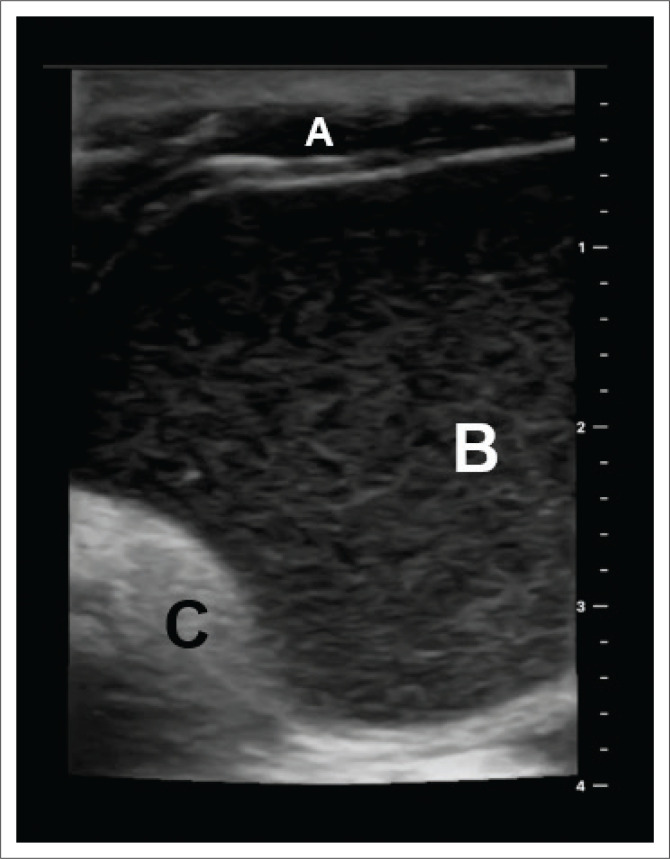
Pre-operative ultrasound imaging. The top of the image is superficial, whereas the bottom of the image is deep. The left of the image approaches the midline, while the right of the image is lateral. Letter ‘A’ marks the anterior belly of digastric. ‘B’ denotes the echogenic material found on the inside of the cyst. ‘C’ marks the posterior acoustic enhancement consistent with cystic structures along the mylohyoid.
